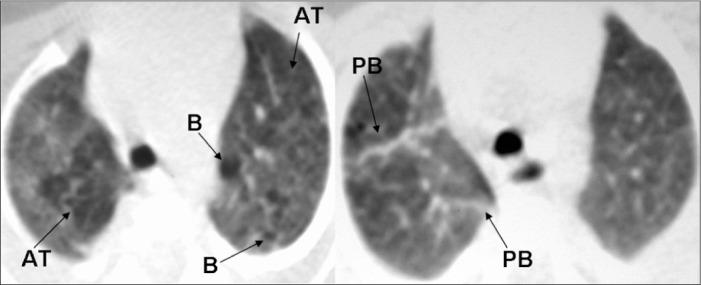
High-resolution computed tomography (HRCT) scan showing air trapping (AT), air bubbles (B) and parenchymal band (PB).
